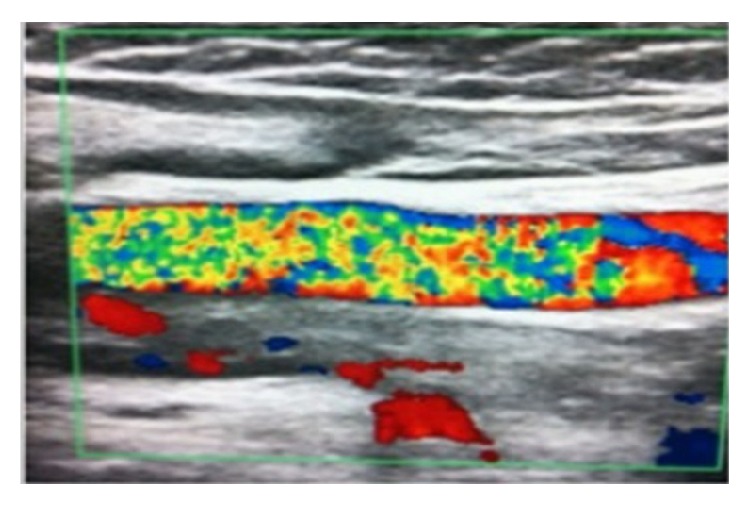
Doppler ultrasound revealed thrombosis in a main femoral vein, 95 × 79 mm.
